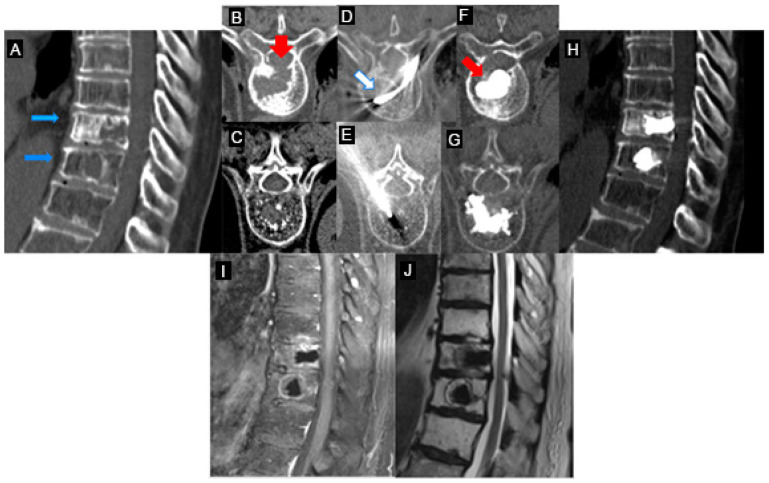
Painful pathological fractures of T8–T9 from lung carcinoma (patient #5-Table 1). (A–C): Axial and sagittal CT images of aggressive destructive osteoblastic metastasis of the T8–T9 vertebral bodies (blue arrows in (A)) with pathological fracture and destruction of the posterior wall of the T8 vertebra (red arrow in (B)). (D): Placement of the STAR™ radiofrequency probe (white arrow) in the central portion of the T8 vertebra lesion. (E,G): Vertebroplasty on the T9 vertebra, given the absence of posterior wall rupture. (F): The Arcadia™ low-compliance, high-pressure balloon was coaxially inserted into the introducer cannula and deployed into the lesion (red arrow). Note the inflation of the low-compliance Arcadia balloon after RFA and the predictable cement distribution. (H–J): Post-vertebroplasty CT (H) and MRI (I,J) sagittal images post procedural control T8 and T9 without complications.
